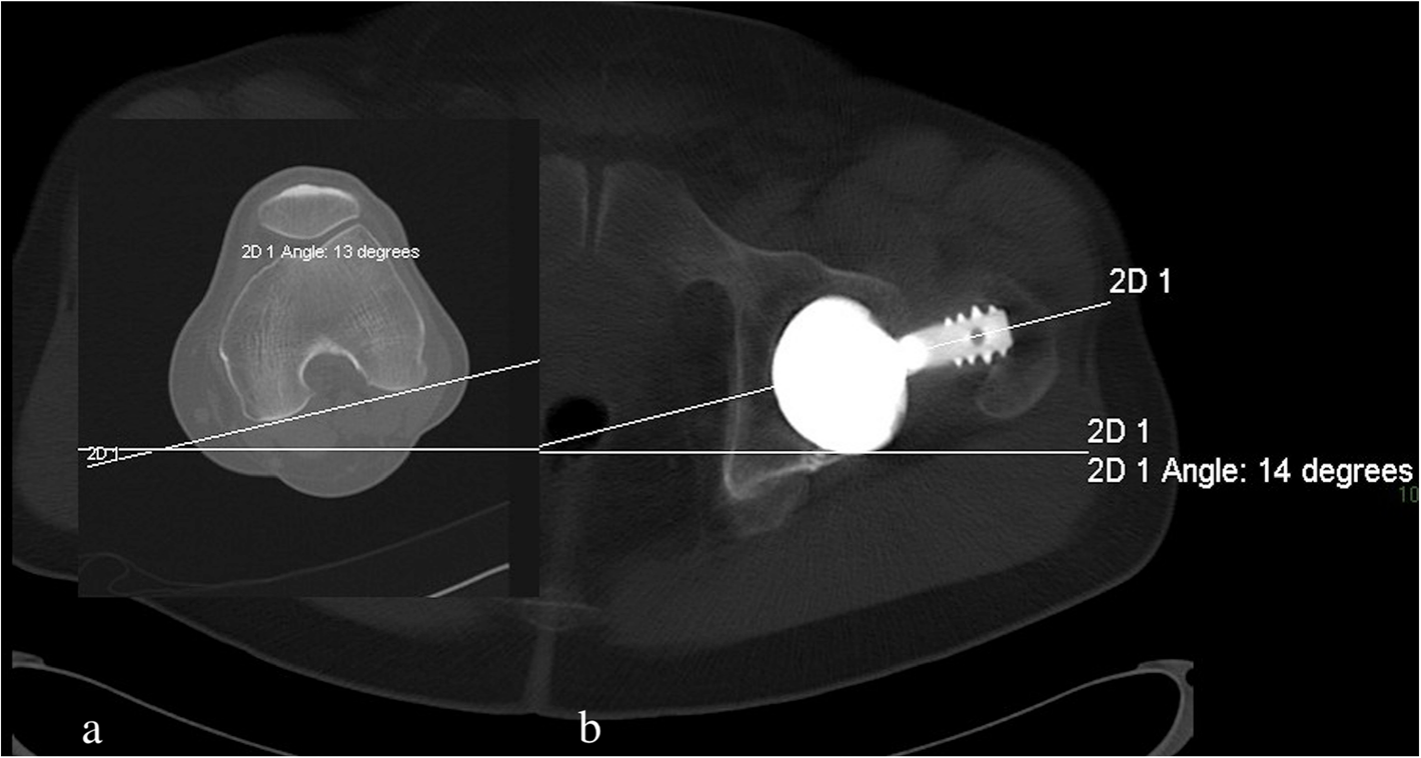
a and b: CT-scan showing 13° of femoral distal condyle rotation and 14° of stem frontal angle. The stem anteversion (1°) is obtained by subtracting the condylar rotation and the stem angle
